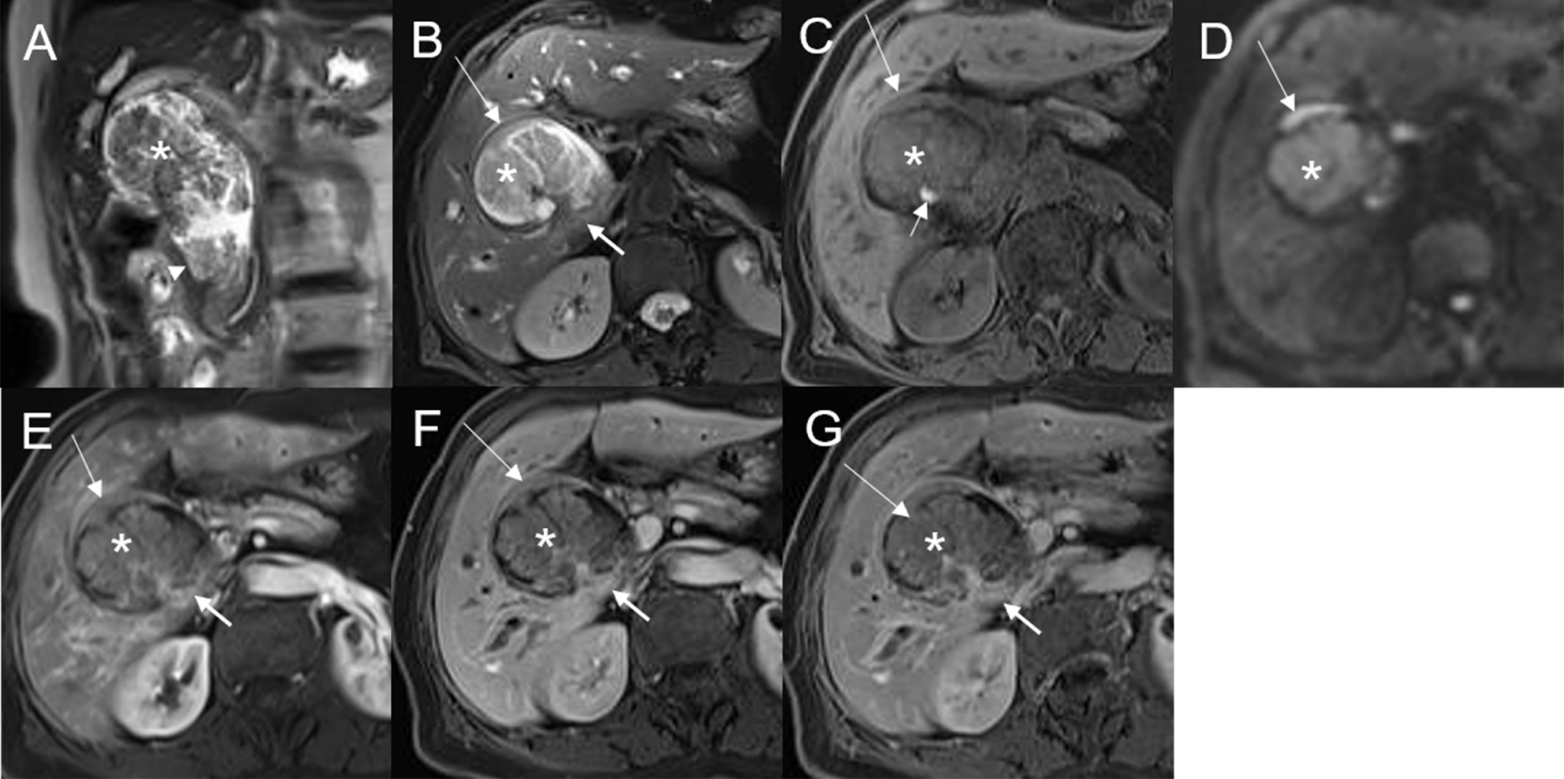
Malignant transformation of adenoma, with three lesions in the common hepatic duct and common bile duct. The first eccentric mass, with a frond-like superficial appearance (*), shows hyperintensity on T2WI (A, B), hypointensity with speckled hyperintensity (short arrow) on the T1WI (C), hyperintensity on DWI (D), and slight continuous enhancement (E–G). The second lesion, presenting as a plaque-like mass (long arrow) shows hyperintensity on T2WI (B), hypointensity on the T1WI (C), hyperintensity on DWI, and hyperintensity on DWI (D). The T1-postcontrast arterial phase (E), portal phase (F), and delayed phase (G) all show slight enhancement. The bile duct wall is thickened at the base of the lesion (thick arrow). The third lesion, presenting as an eccentric mass (arrow), is hyperintense on coronal T2WI (A). The bile duct upstream and at the site of the most distal lesion is seen dilated on coronal T2WI (A).
